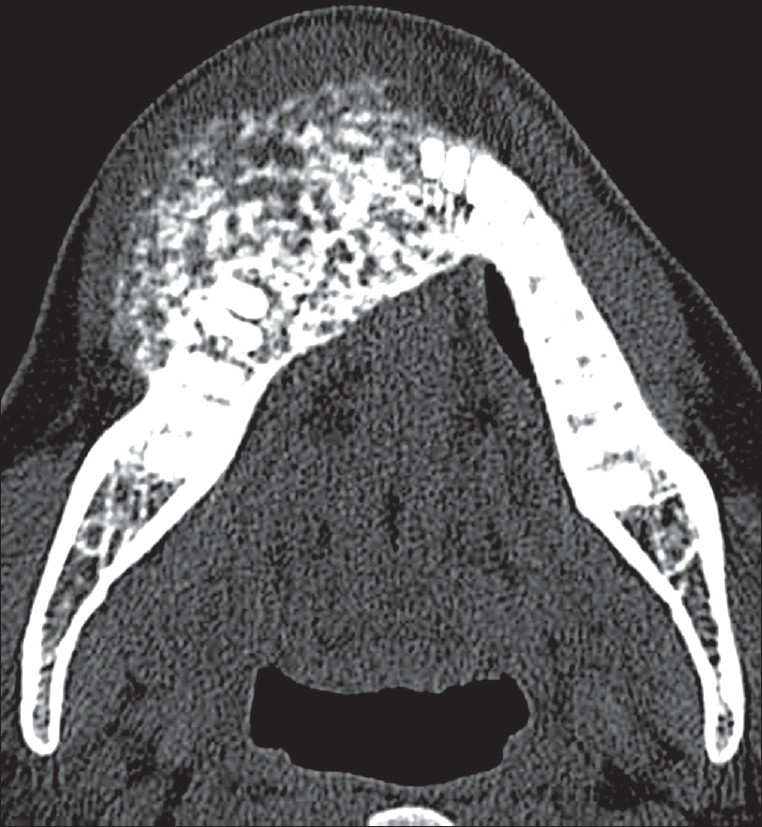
Axial CT of the mandible with bone window settings, shows an expansile, solid, mixed radiodense-radioopaque lesion with poorly defined margins on the right anteriorly, with destruction of the cortices and extension into the soft tissues and across the symphysis as well
